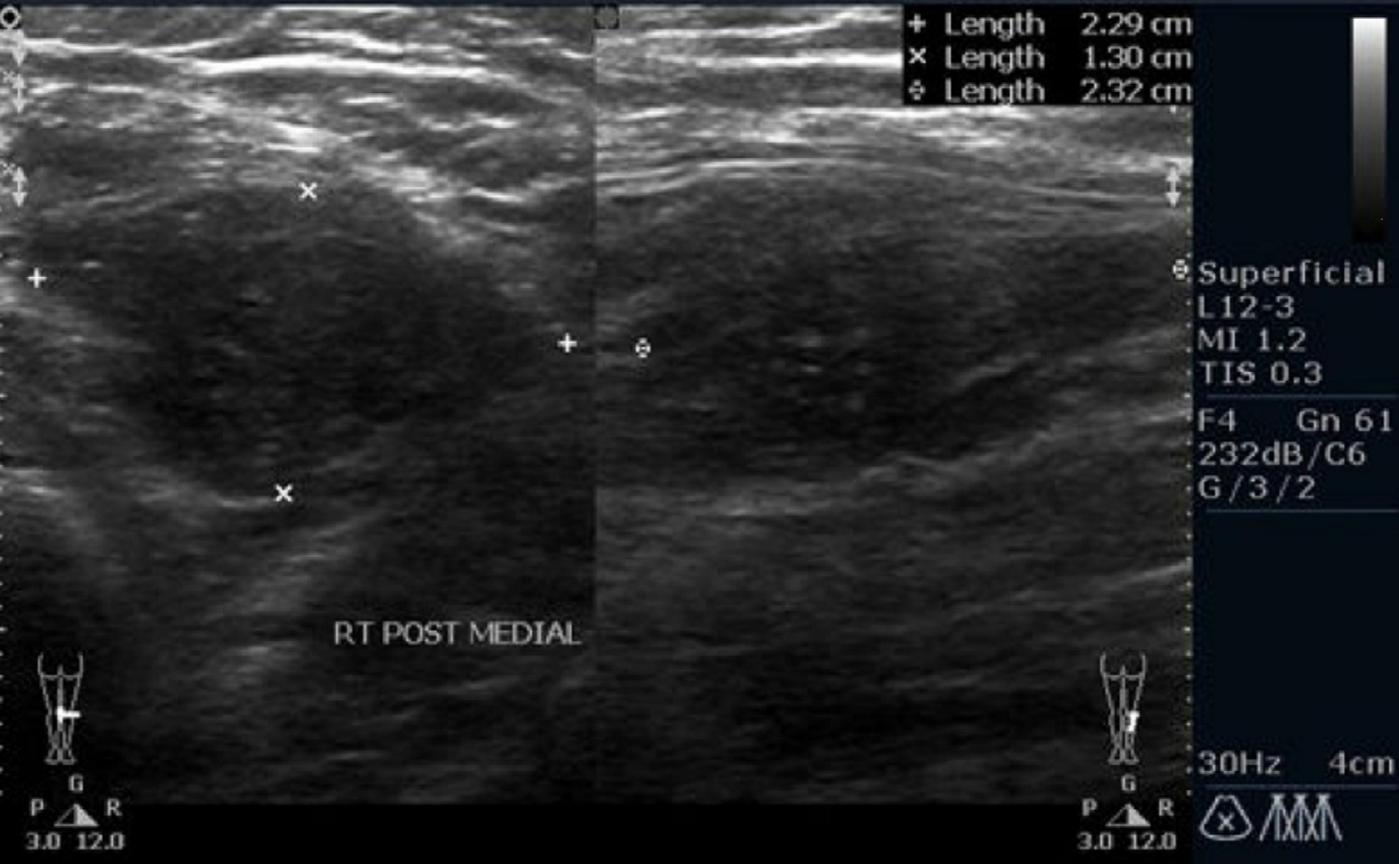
Ultrasonography of right leg. Hypoechoic lesion (abscess) at subcutaneous tissue of posteromedial aspect of the right leg.
